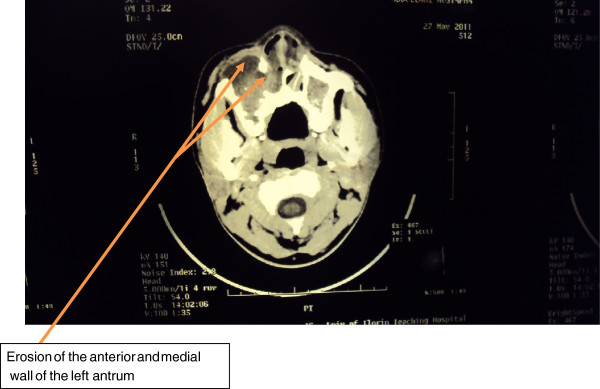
Computed tomography scan (axial) of our patient, showing the isodense lesion within the right maxillary antrum and slight erosion of the anterior and medial walls.
